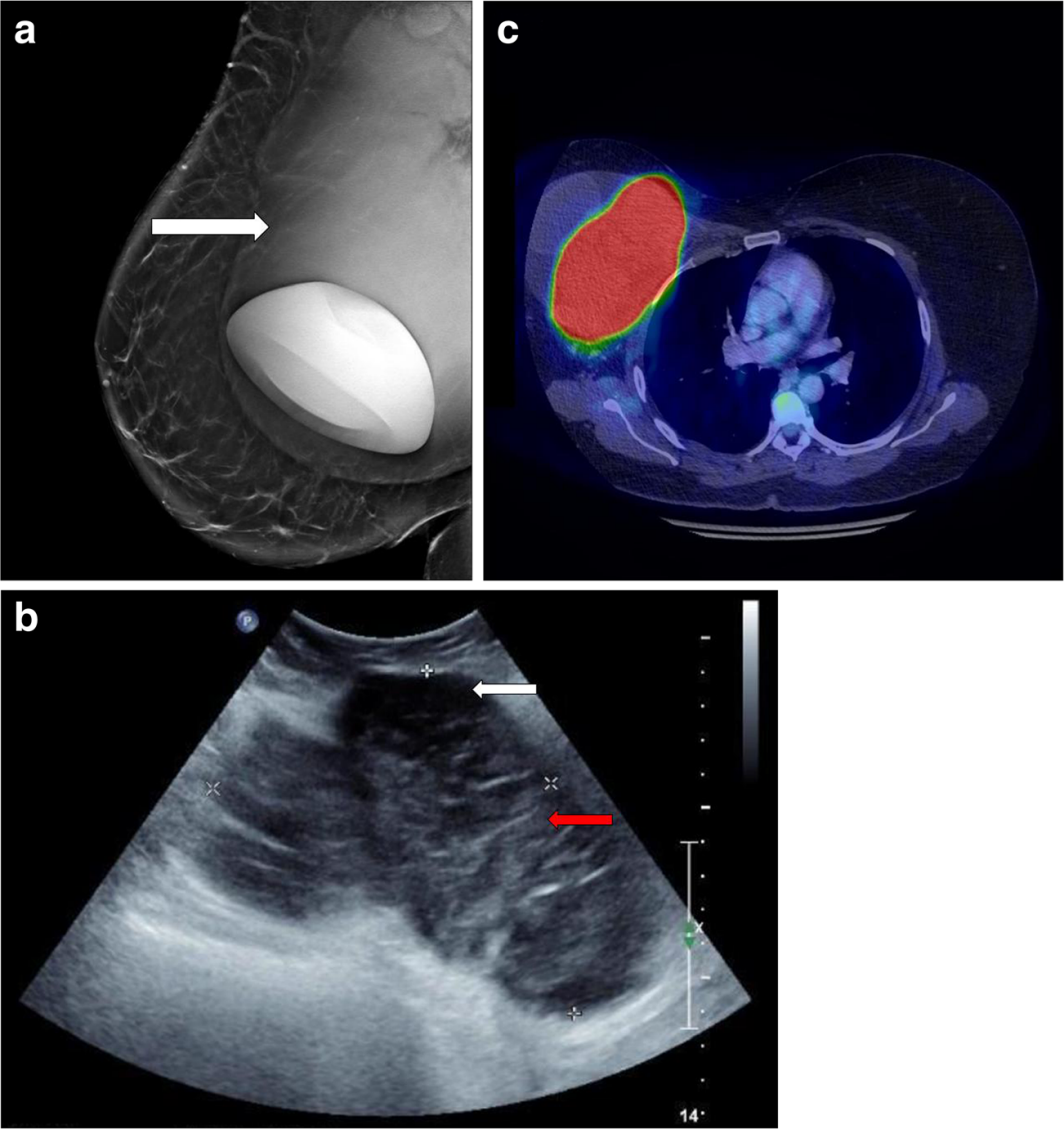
a The mammogram revealed that the implant was displaced anteriorly and inferiorly by a large, lobular, ill defined, soft tissue density mass (*white arrow*). The implant appears intact but compressed. b Ultrasound revealed a peri-implant effusion (*white arrow*), with the implant displaced and compressed by a large lobular solid heterogeneous mass (*red arrow*). Masses, as in this case, are unusual in breast implant-associated anaplastic large cell lymphoma (BIA-ALCL). The diagnosis was confirmed by core biopsy of the mass. c Positron emission tomography/computed tomography (PET/CT) revealed a large mixed-density mass with intense FDG activity, deep within and invading the right breast and pectoralis muscles. There was metastatic disease spread to the lung and bone
